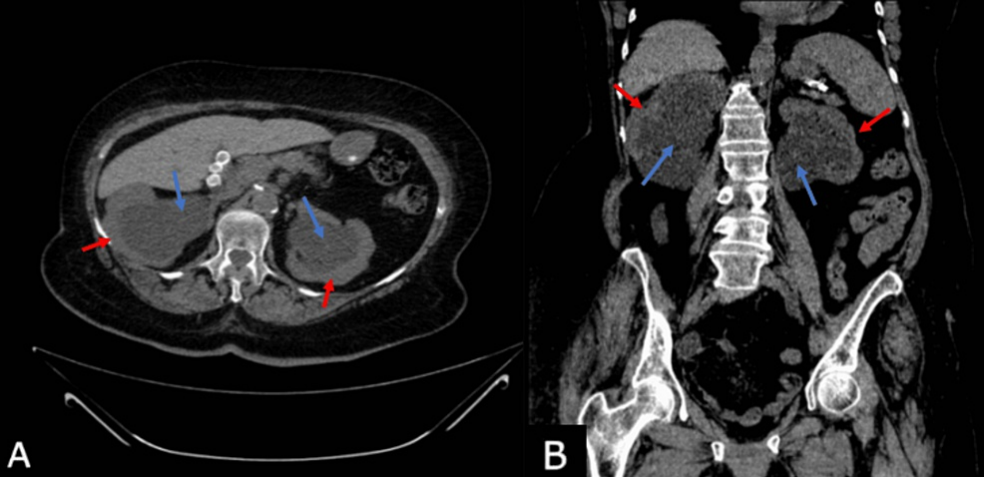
CT scans of the abdomen; coronal and axial views. Axial and coronal CT scans of the abdomen without IV contrast (A and B) demonstrating severe bilateral hydronephrosis (blue arrows) with thinning of the renal cortex bilaterally (red arrows).
